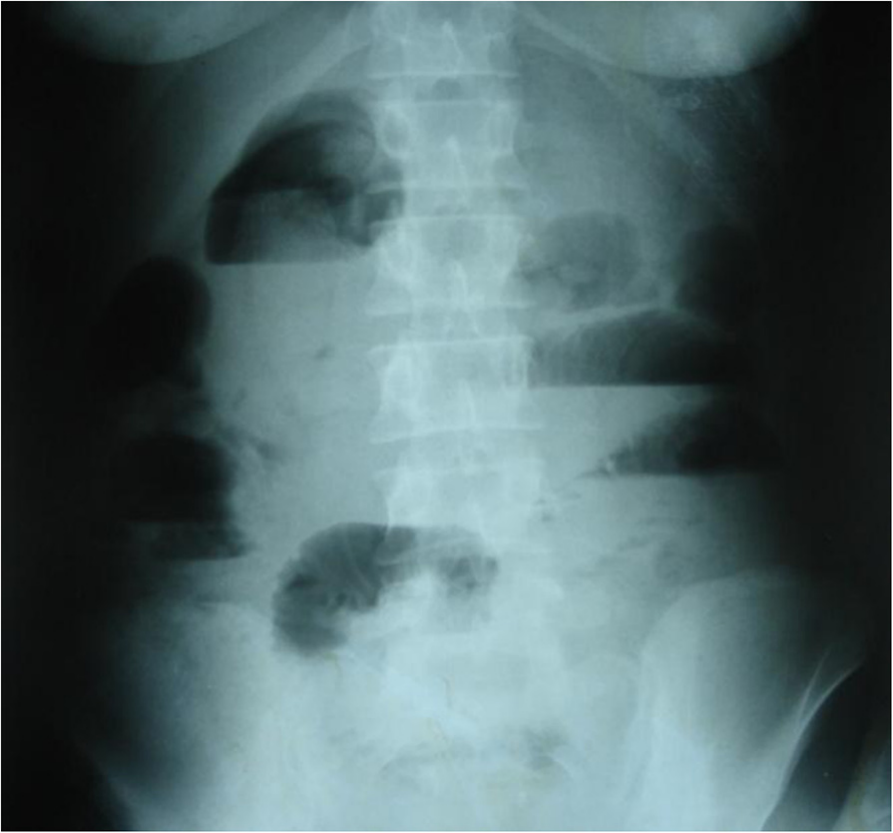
Upright plain abdominal X-ray demonstrating a small bowel obstruction. Note the presence of multiple air fluid levels.
